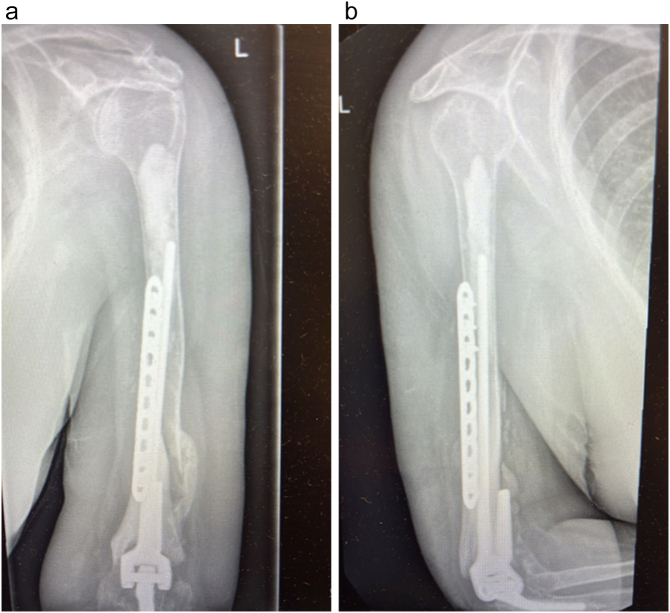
Post-operative X-ray and one month follow up. In anteroposterior [A] and lateral [B] views the area around the humeral stem is stable with no loosening present or any early resorption of the allograft.
